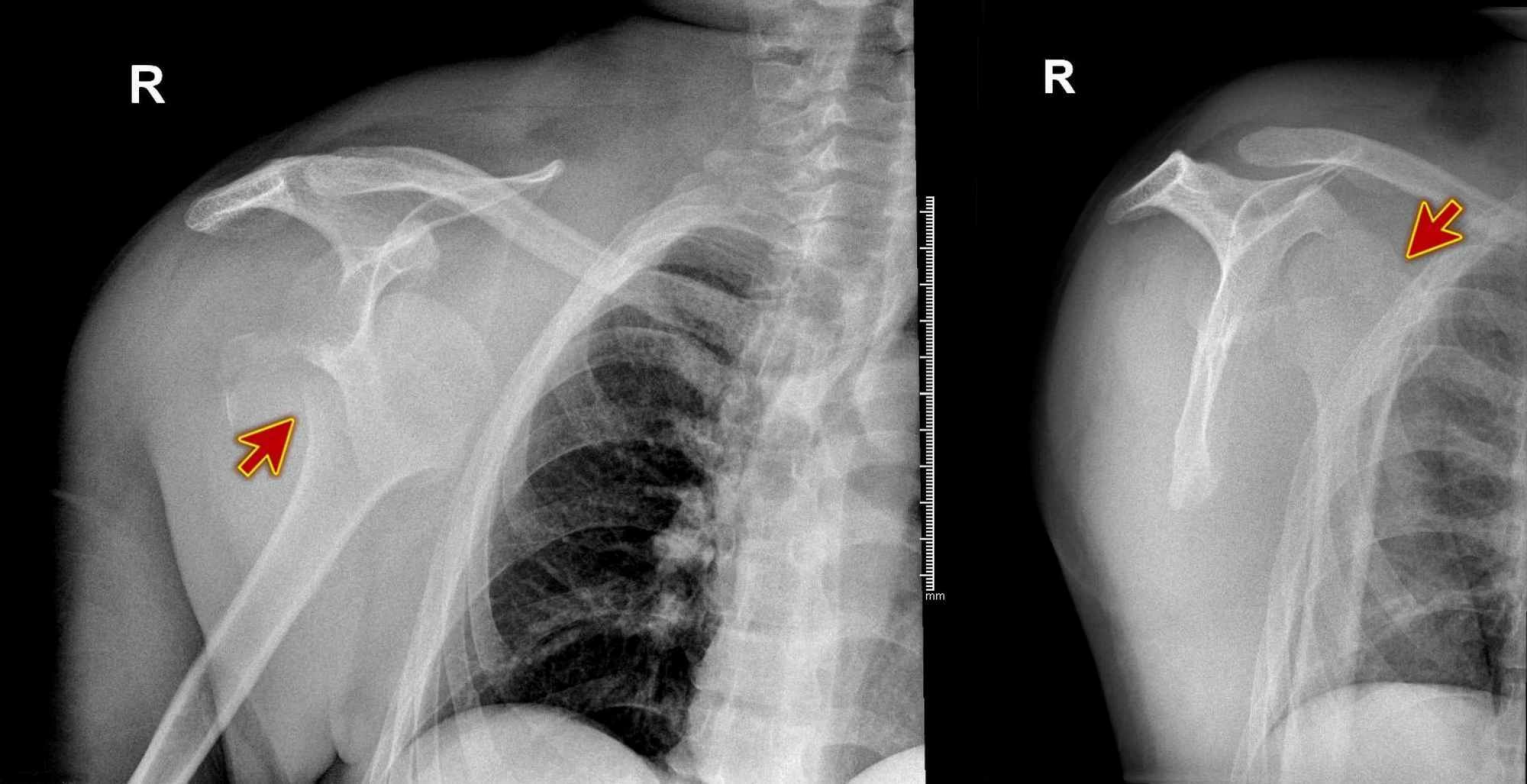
X-ray of the right shoulder showing anterior dislocation and fracture of the humeral head.
